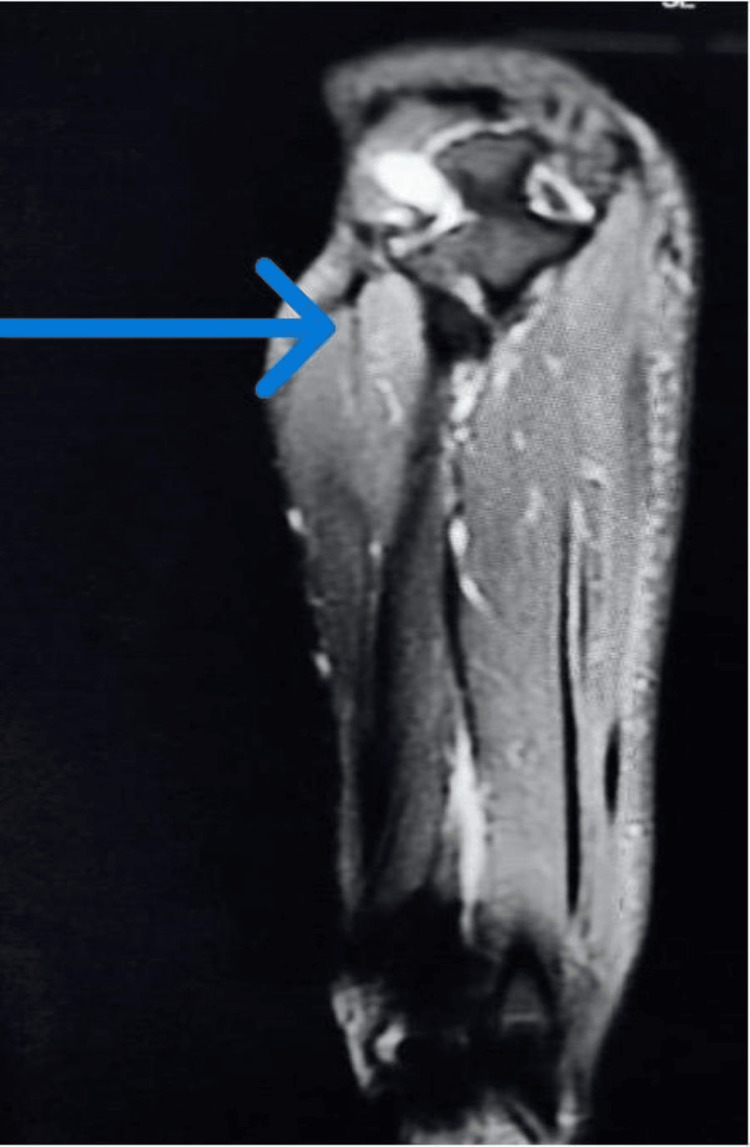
MRI right forearm Hyperintense edematous signal in subcutaneous plane, cubital fossa region surrounding underlying vessels, and mild joint effusion in elbow.
